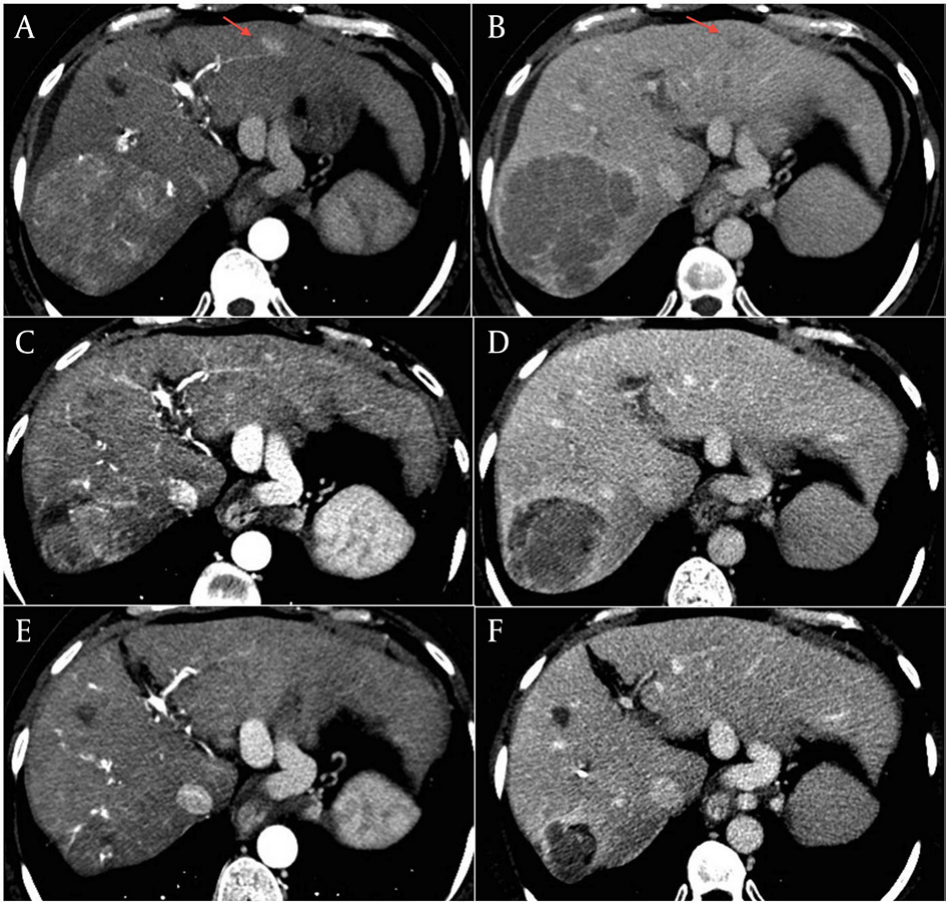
Contrast-enhanced CT scan of the liver performed as baseline assessment in March 2012 (A and B), at the end of June 2012 (C and D), and in January 2013, showing a progressive shrinkage of the the large HCC lesion of the right lobe. A, C, and E panels: arterial phase. B, D, and F panels: equilibrium phase Note that the HCC lesion detected in the segment II (arrows in panels A and B) was also markedly reduced at the first CT control (C and D), and was no longer clearly distinguishable from the surrounding tissue at the last radiologic control (E and F).
